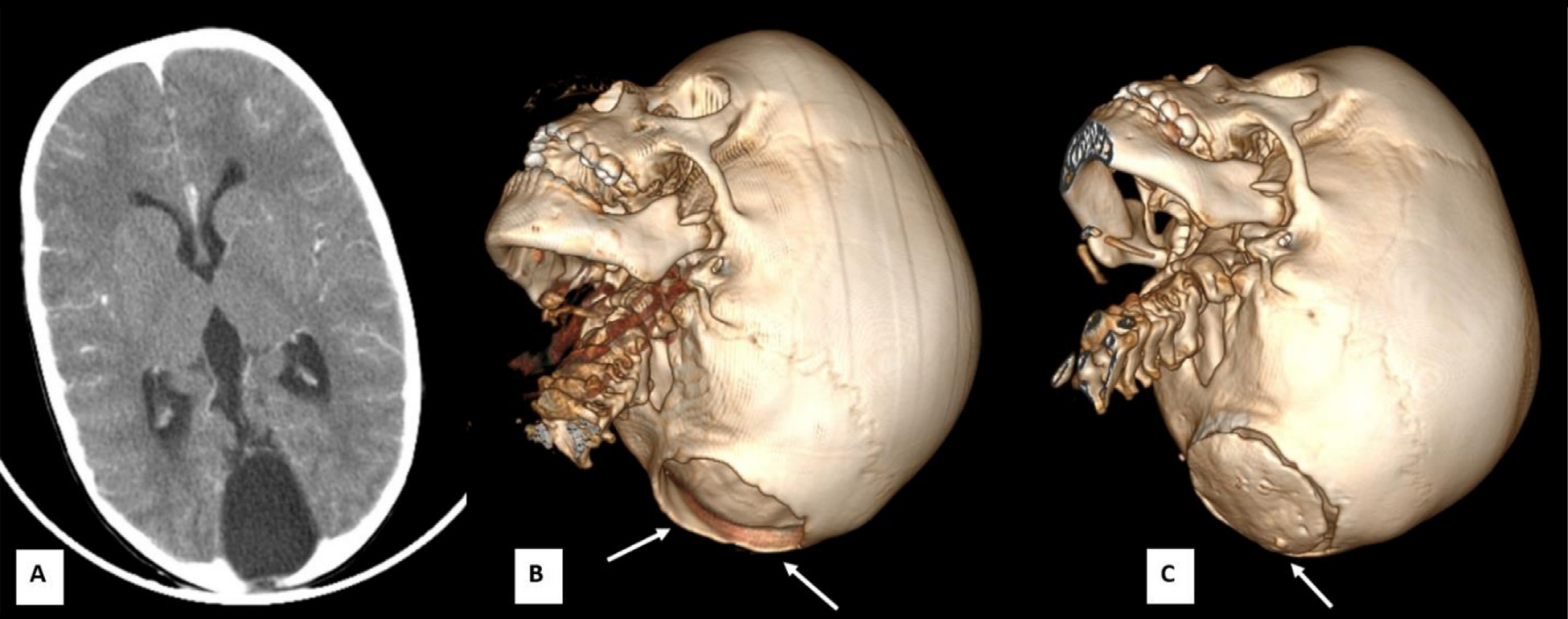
Joubert plus syndrome with posterior fossa cyst and occipital bone defect. (A) Axial contrast-enhanced CT image demonstrates a CSF-filled cystic structure in the posterior fossa, causing smooth remodeling and thinning of the surrounding occipital bone with focal dehiscence. (B) Preoperative 3D volumetric CT reconstruction shows a 6 × 3 cm bone defect in the midline occipital region (solid white arrows). (C) Postoperative 3D volumetric CT image reveals placement of a cranioplasty bone flap over the defect site (solid white arrow).
